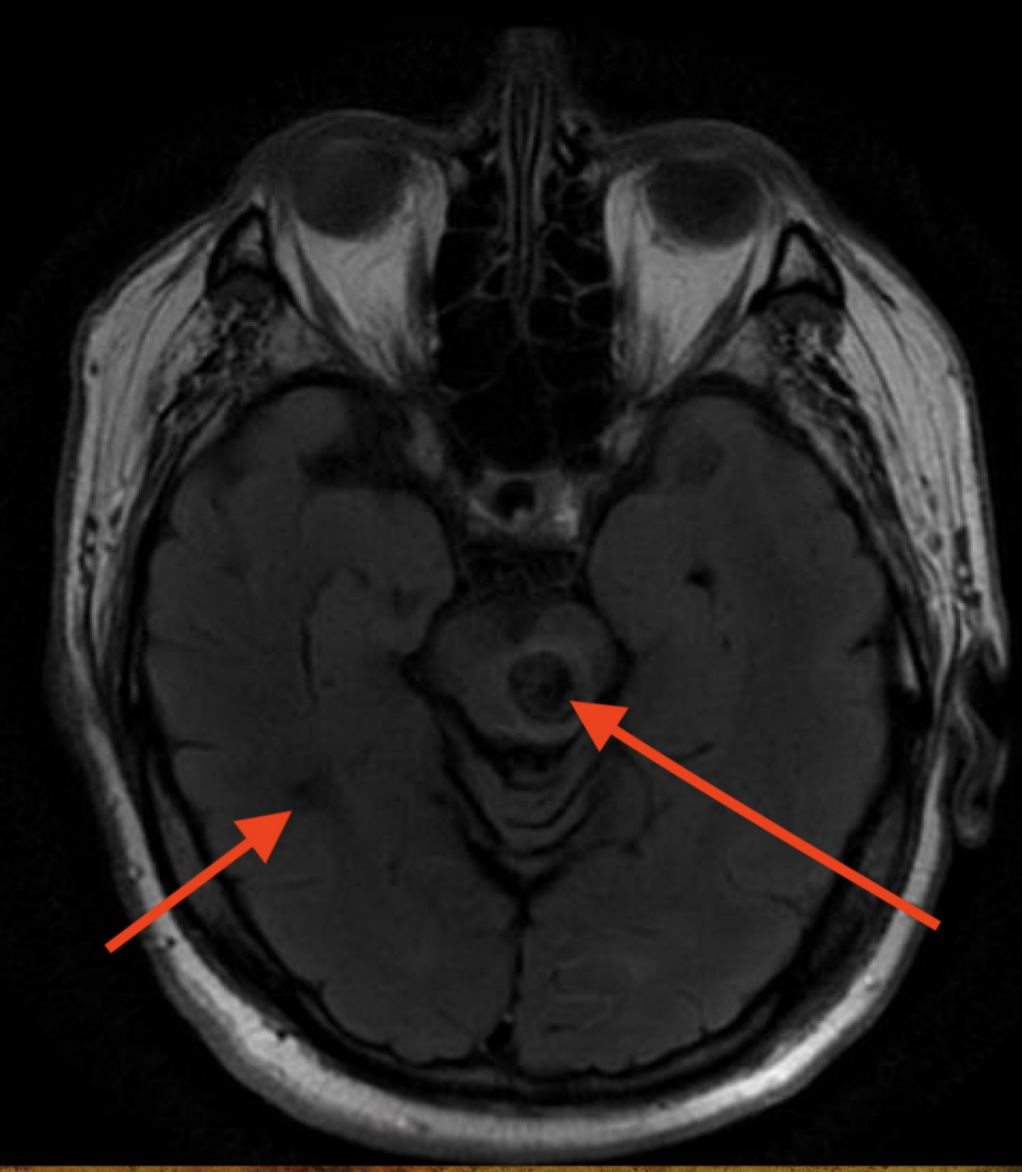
MRI FLAIR showing large hemosiderin depositions in the areas of cavernomas. FLAIR: Fluid-attenuated inversion recovery
